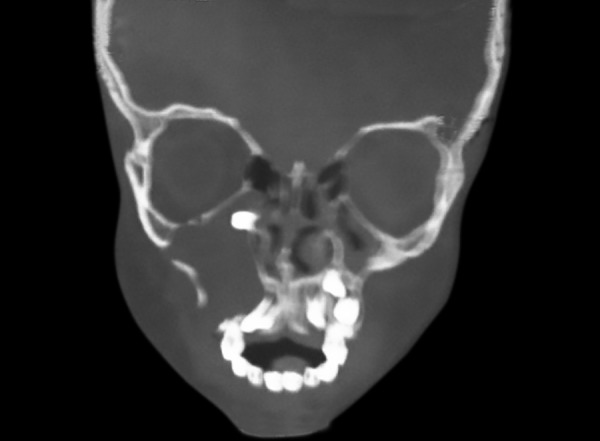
Computed tomography coronal view showing cystic occupying maxillary sinus and tooth bud displaced close to the orbital floor
